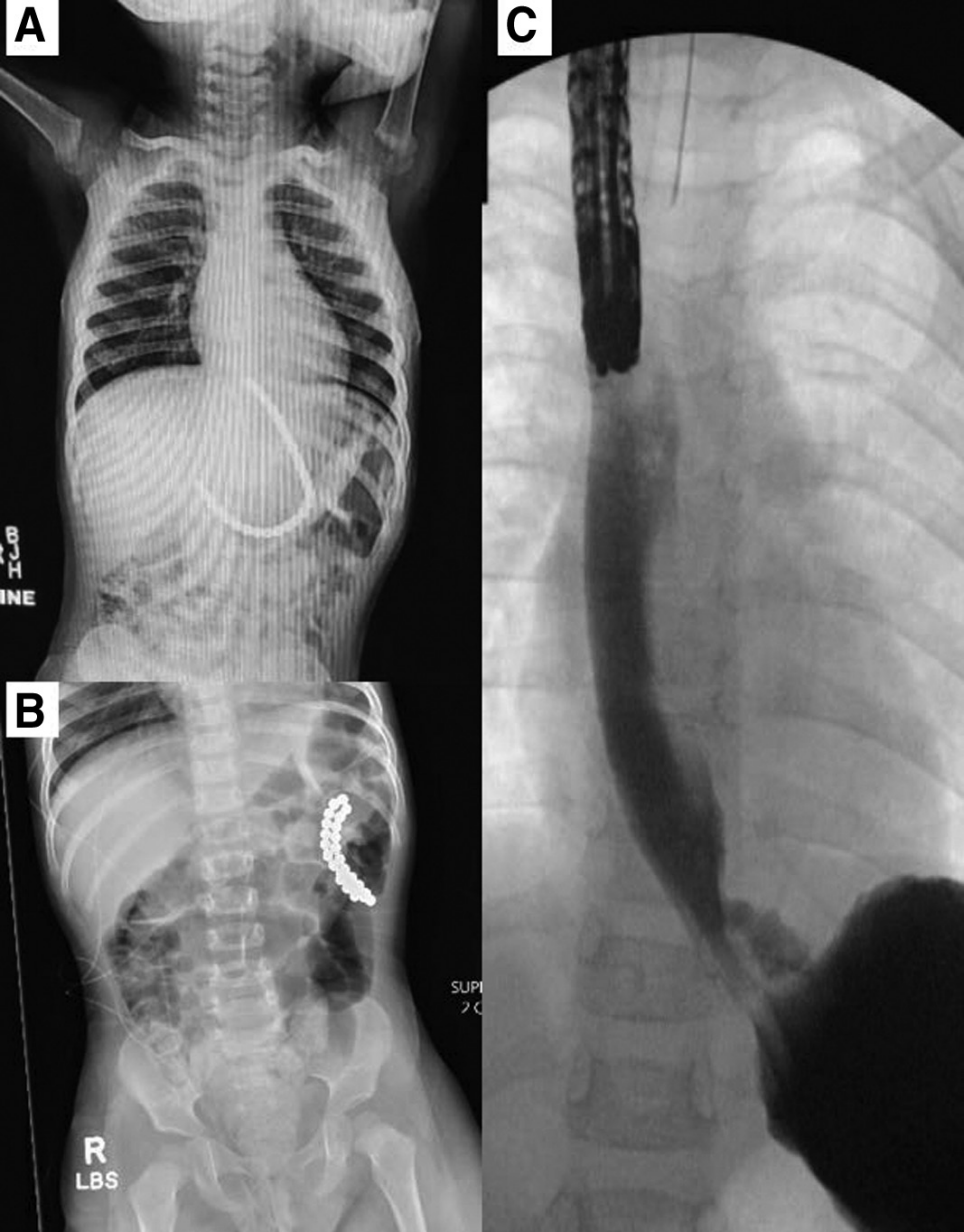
Radiographic and contrast studies showing (A) magnets lining the esophagus to the duodenum, (B) magnets looped in the stomach and (C) esophagogastic fistula created.
